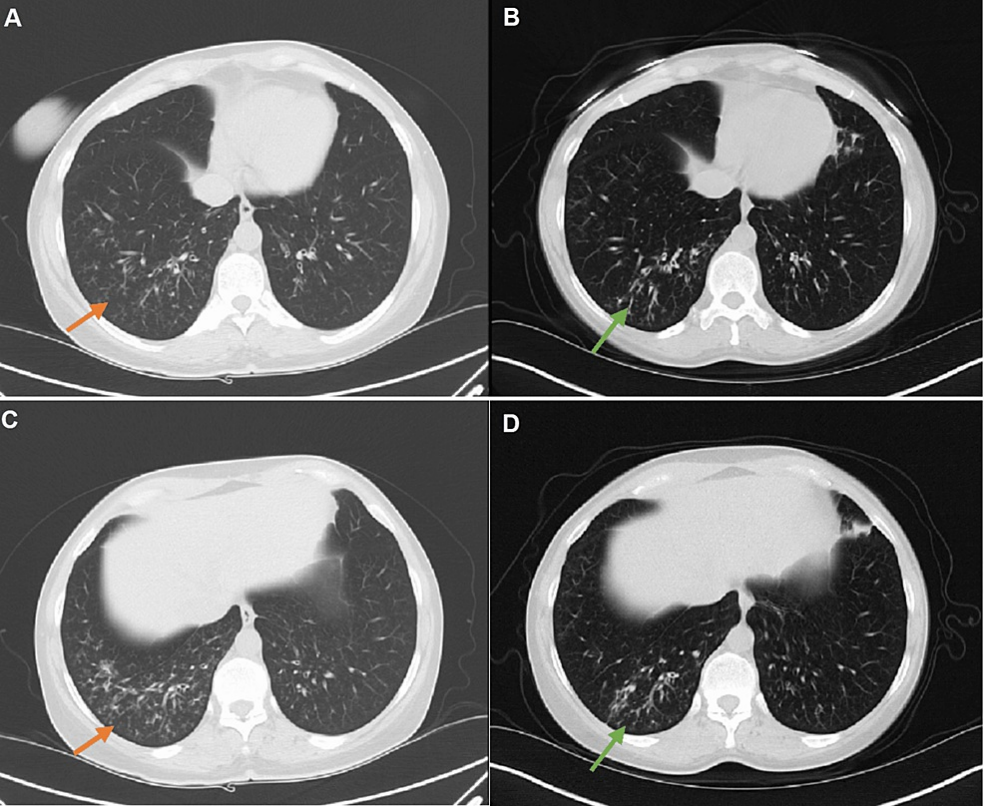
Regression of pulmonary nocardiosis during the treatment course from May 2022 to December 2022. (A, C) CT chest from May 2022 revealed mild linear opacities mostly in the right lower lobe; (B, D) Follow-up CT chest in December 2022 after seven months of treatment with TMP-SMX revealed bibasilar peribronchial thickening and tree-in-bud nodularity slightly increased in the right lower lobe and decreased in the left lower lobe. TMP/SMX: trimethoprim/sulfamethoxazole
